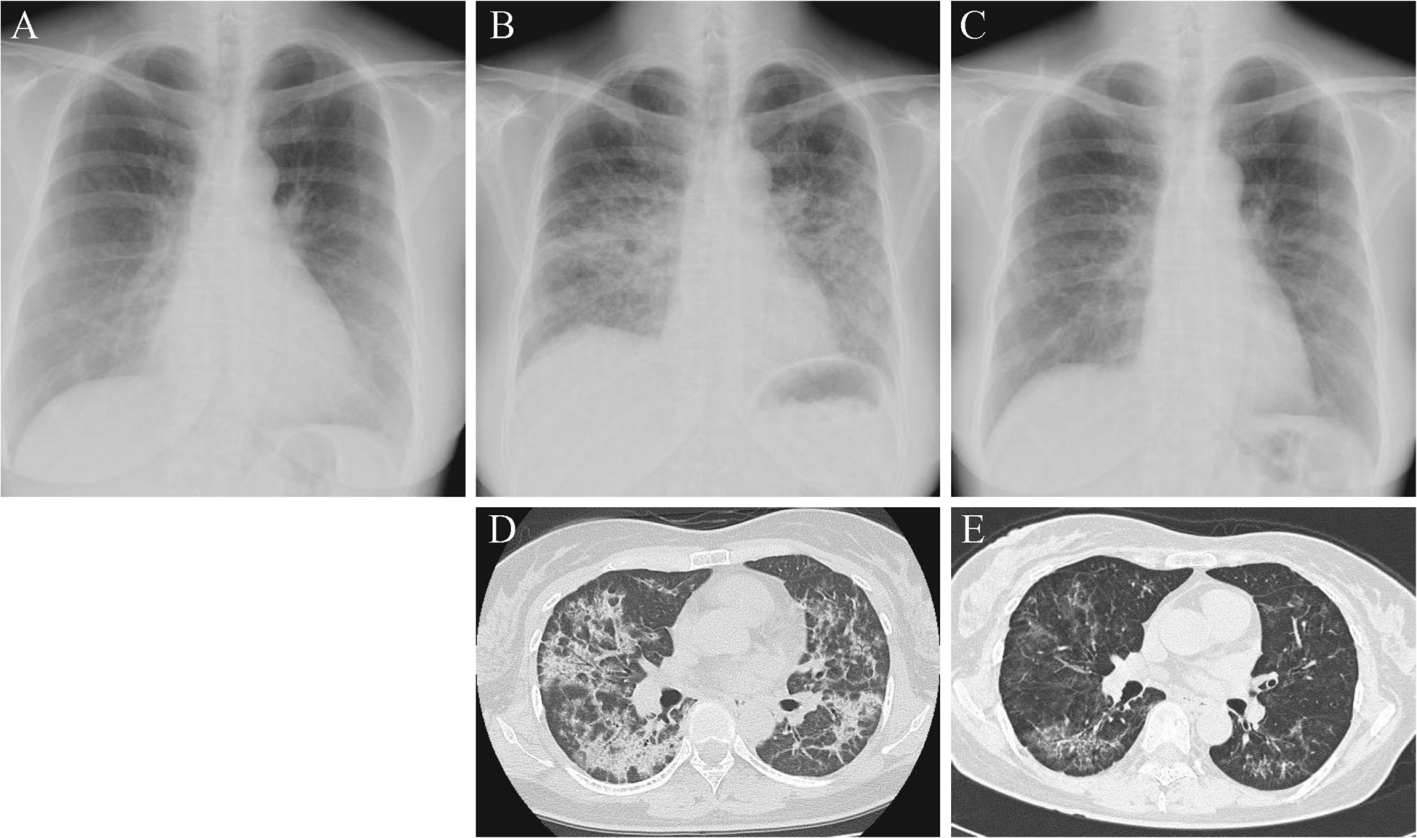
Images of the patient’s lungs. Chest radiography performed on the day imatinib was discontinued (A) showed no abnormal findings. Chest radiography on admission (B) revealed bilateral reticular shadows in both mid and lower lung fields. Chest high-resolution computed tomography (HRCT) scans obtained on admission (D) revealed diffuse interstitial infiltrates in both lungs, predominantly along the bronchovascular bundles extending to subpleural regions. Two months after corticosteroid therapy, chest radiography (C) and HRCT scans (E) indicated remarkable improvement.
